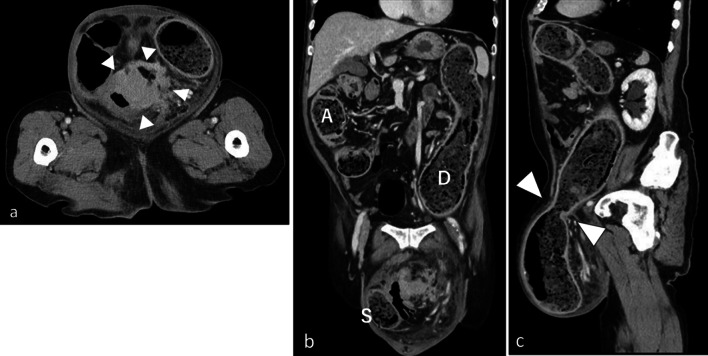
CT in the 2nd hospital days Wall thickening (arrow head) of the incarcerated intestine within the hernia was shown at axial view (a). Large amounts of faces in the ascending (A), descending (D) and sigmoid colon within the hernia (S) at coronal view (b). An enlarged hernia orifice (arrow head) was shown at sagittal view (c)
